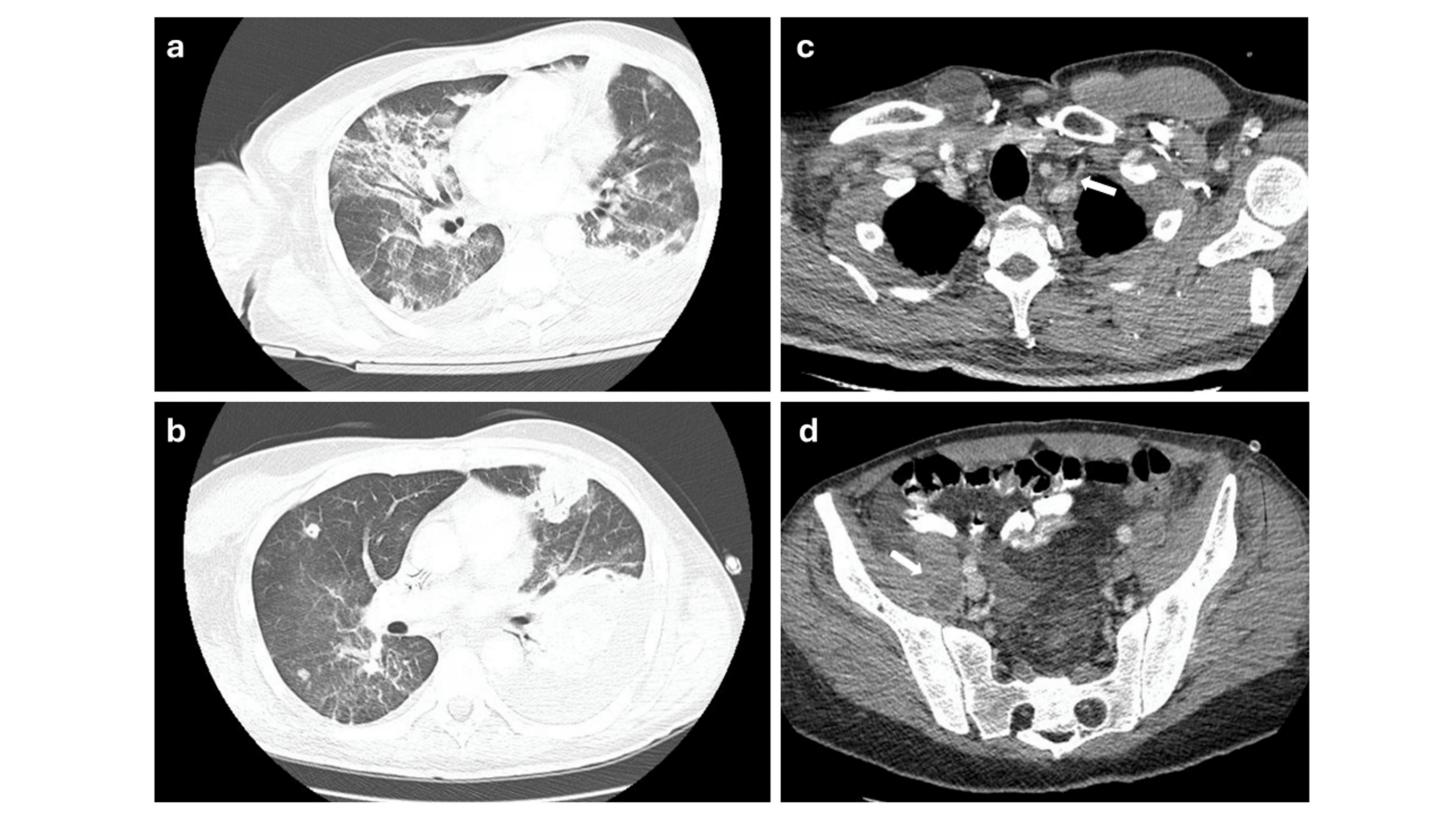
Thorax CT scan at presentation (a) and two weeks later (b). Bilateral pulmonary infiltrates and ground-glass opacities (a). Development of nodular lesions and a left parapneumonic fluid collection (b). Thrombosis of the left internal jugular vein (white arrow) (c). Abscess of the right iliopsoas muscle (white arrow) (d).
